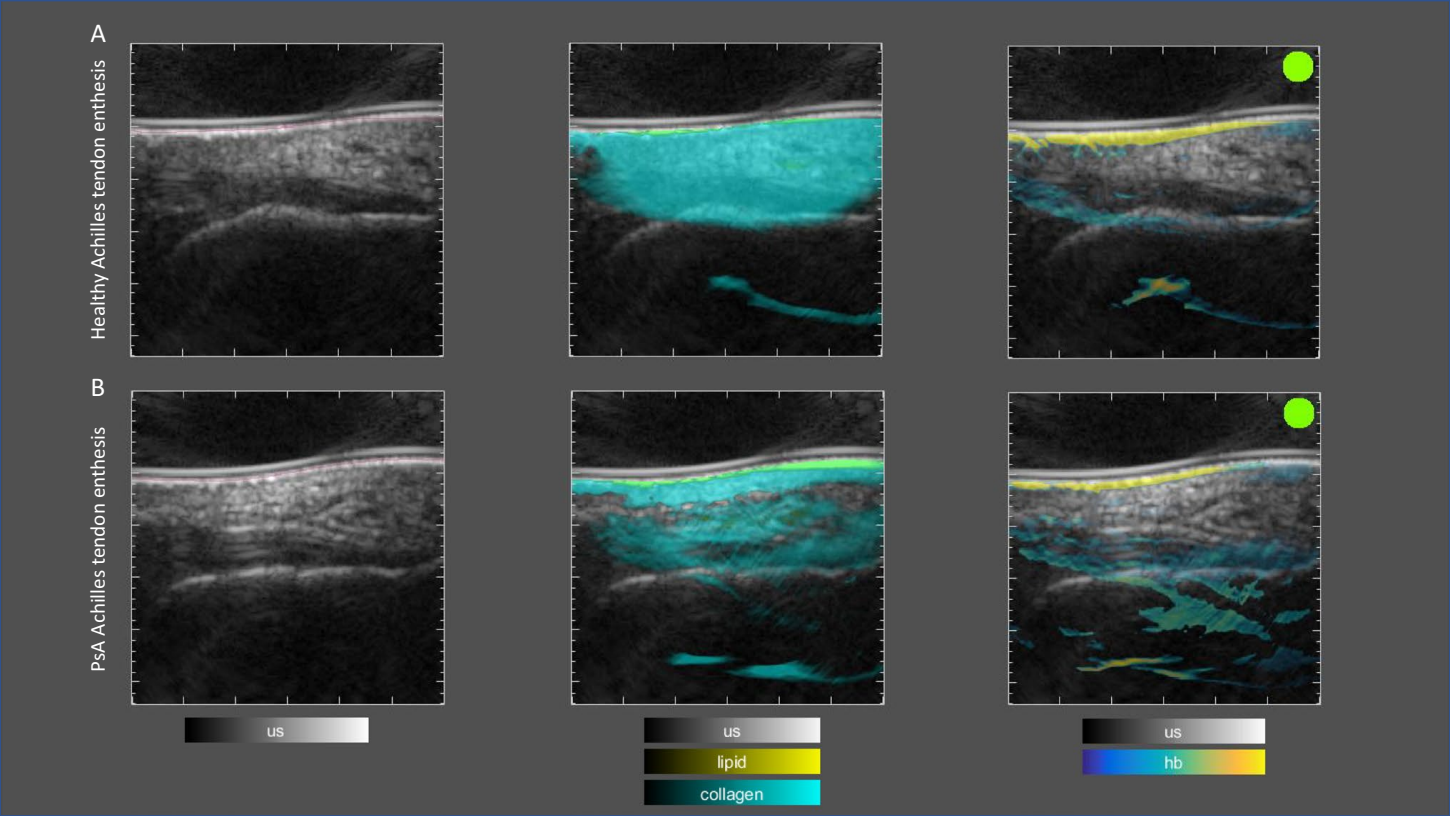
MSOT imaging of the Achilles tendon entheses of a patient with active PsA. The images show normal findings in the left Achilles tendon (A, upper panels) and signs of enthesitis of the right Achilles tendon (B, lower panels). The healthy enthesis shows normal ultrasound findings (left panel), homogeneous collagen distribution (middle panel), and no visible increase in hemoglobin (right panel). The inflamed enthesis (B, lower panels) was clinically tender, swelling was visible on ultrasound (left panel), collagen was reduced and inhomogeneously distributed (middle panel), hemoglobin was diffusely increased and especially at the entheseal site (right panel). US: ultrasound; hb: hemoglobin
